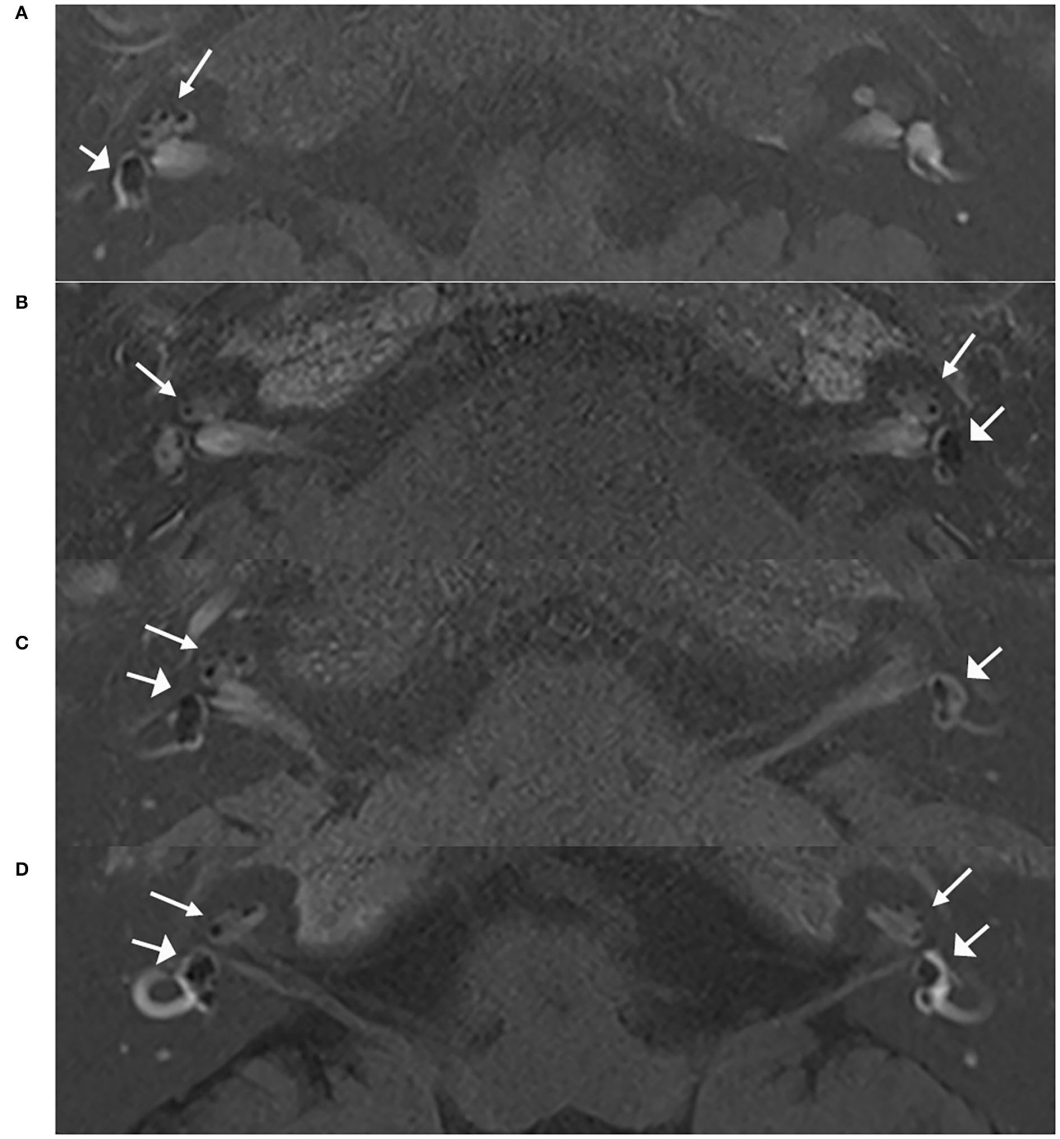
MRI scans of patients with unilateral and bilateral ELH. Images of 3D real IR performed 4 h after intravenous Gd injection. (A) Vestibular and cochlear ELH on the right side in a patient with unilateral MD; (B) Vestibular and cochlear ELH on the left side and cochlear ELH on the contralateral side in a patient with unilateral MD; (C) Vestibular and cochlear ELH on the right side and vestibular ELH on the contralateral side in a patient with unilateral MD; (D) Vestibular and cochlear ELH on the bilateral ears in a patient with bilateral MD. MD, Ménière's disease. Cochlea (thin arrows) and vestibule (broad arrows).
